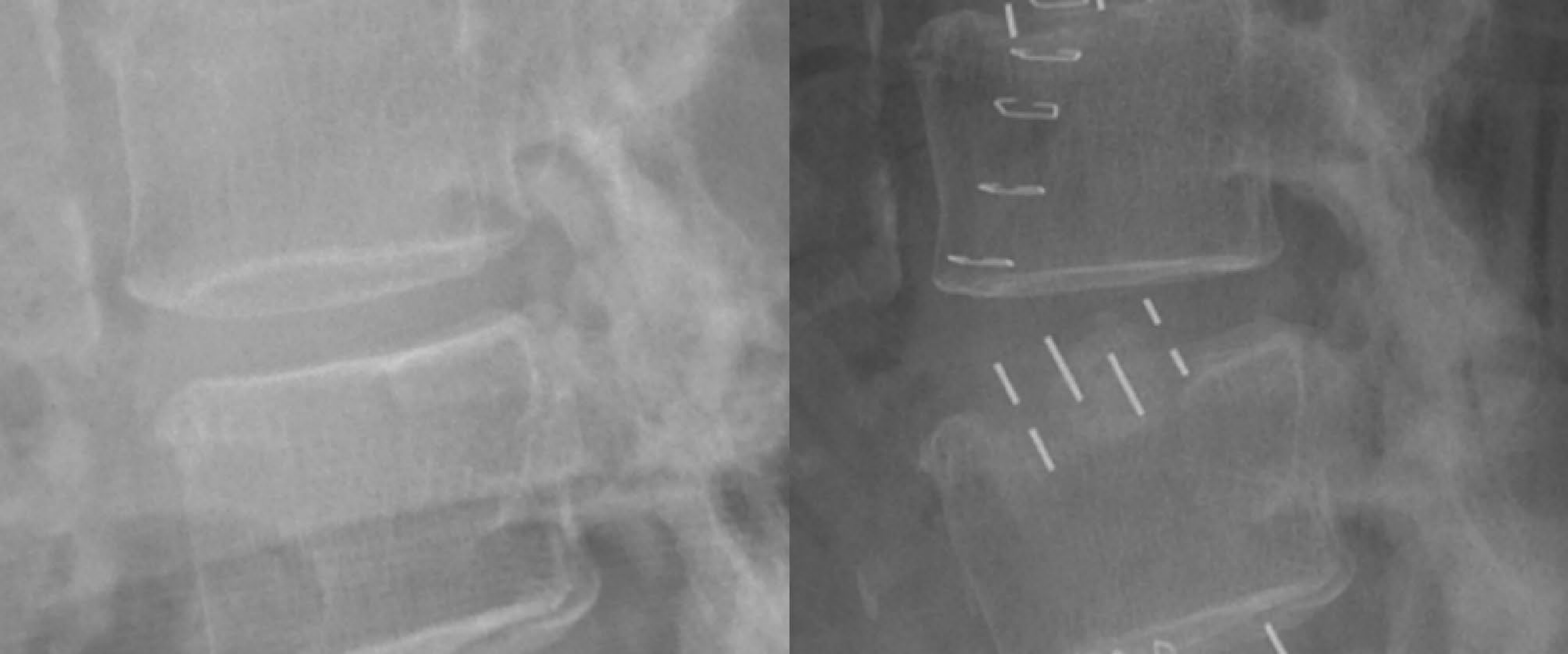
Intraoperative endplate injury during lateral lumbar interbody fusion can be identified by comparison between the preoperative (left) and postoperative (right) X-rays.
